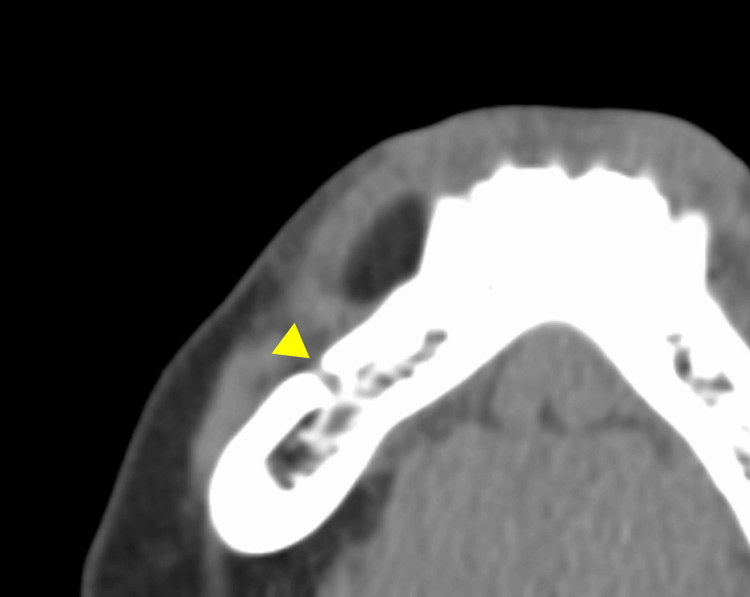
CT image Axial CT image showing a well-circumscribed, homogeneous low-density lesion measuring 17 × 7 × 10 mm in the right mandibular alveolar region. The lesion is located approximately 3 mm anterior to the mental foramen (arrow).
